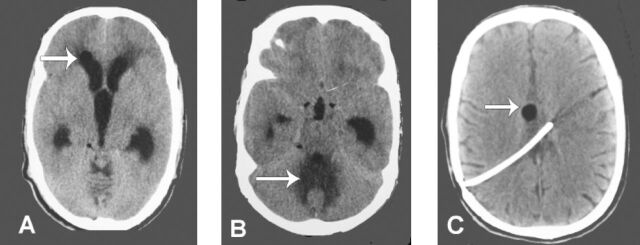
Pre-op non-contrast CT brain a) Features of hydrocephalus with marker showing fat in lateral ventricle, b) Changes in the intensity in vermis, possible site of dermoid cyst after rupture and C) Post-op CT scan decompressed ventricle post–operatively with shunt in situ and marker showing fat.
